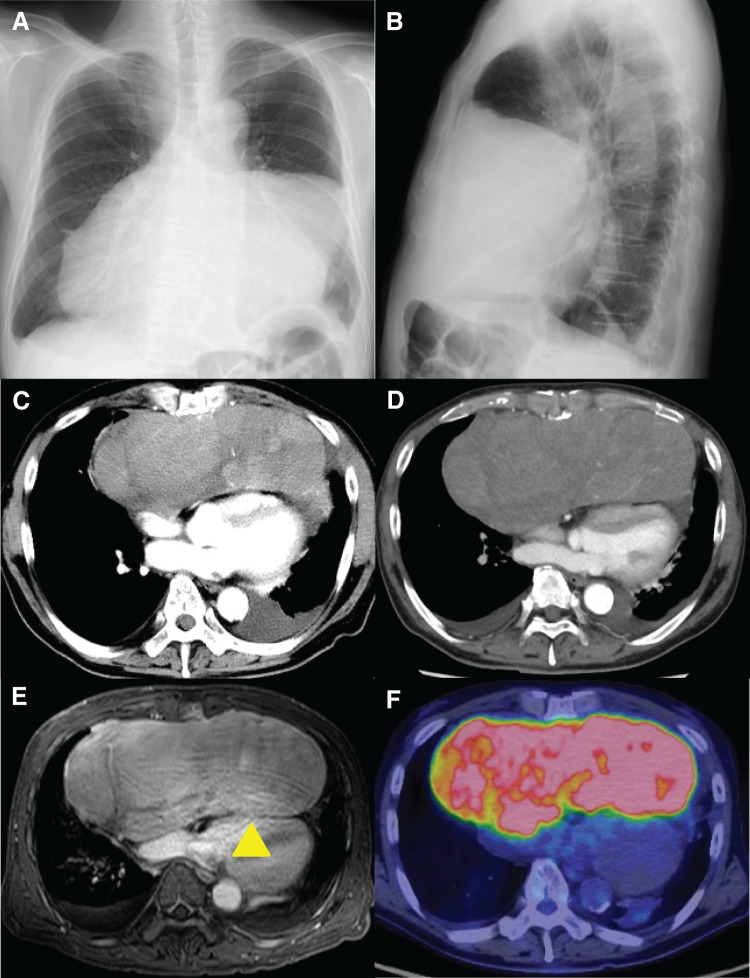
Chest X-ray shows enlarged anterior mediastinal shadow (A: posteroanterior view; B: lateral view). Chest contrast-enhanced CT (C: 39 days before the surgery; D: 4 days before the surgery) scan confirms a rapidly growing, giant lobulated and contrast-enhanced tumor of the anterior mediastinum, measuring 18 × 13 × 7 cm (C) with cardiac compression and extension into both pleural cavities. CT image 4 days before the surgery shows that the tumor presented a rapid growth to 22 × 14.5 × 8.5 cm (D). Contrast-enhanced magnetic resonance imaging suggests tumor invasion to the left anterior chest wall and pericardium close to the right atrium (E, arrowhead). PET-CT reveals increased uptake of fluorodeoxyglucose in the mass (maximum standardized uptake value = 21.5) (F).
